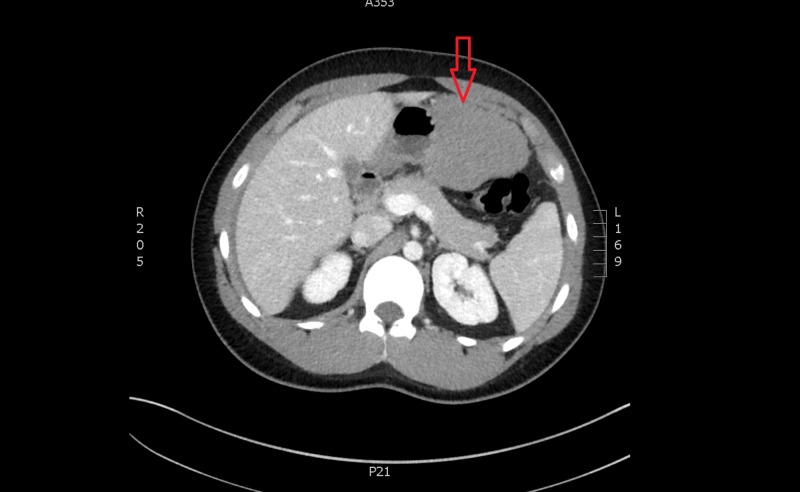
Computed tomography of the abdomen (axial) showing a heterogeneous mass from the greater curvature of the stomach (see red arrow)
